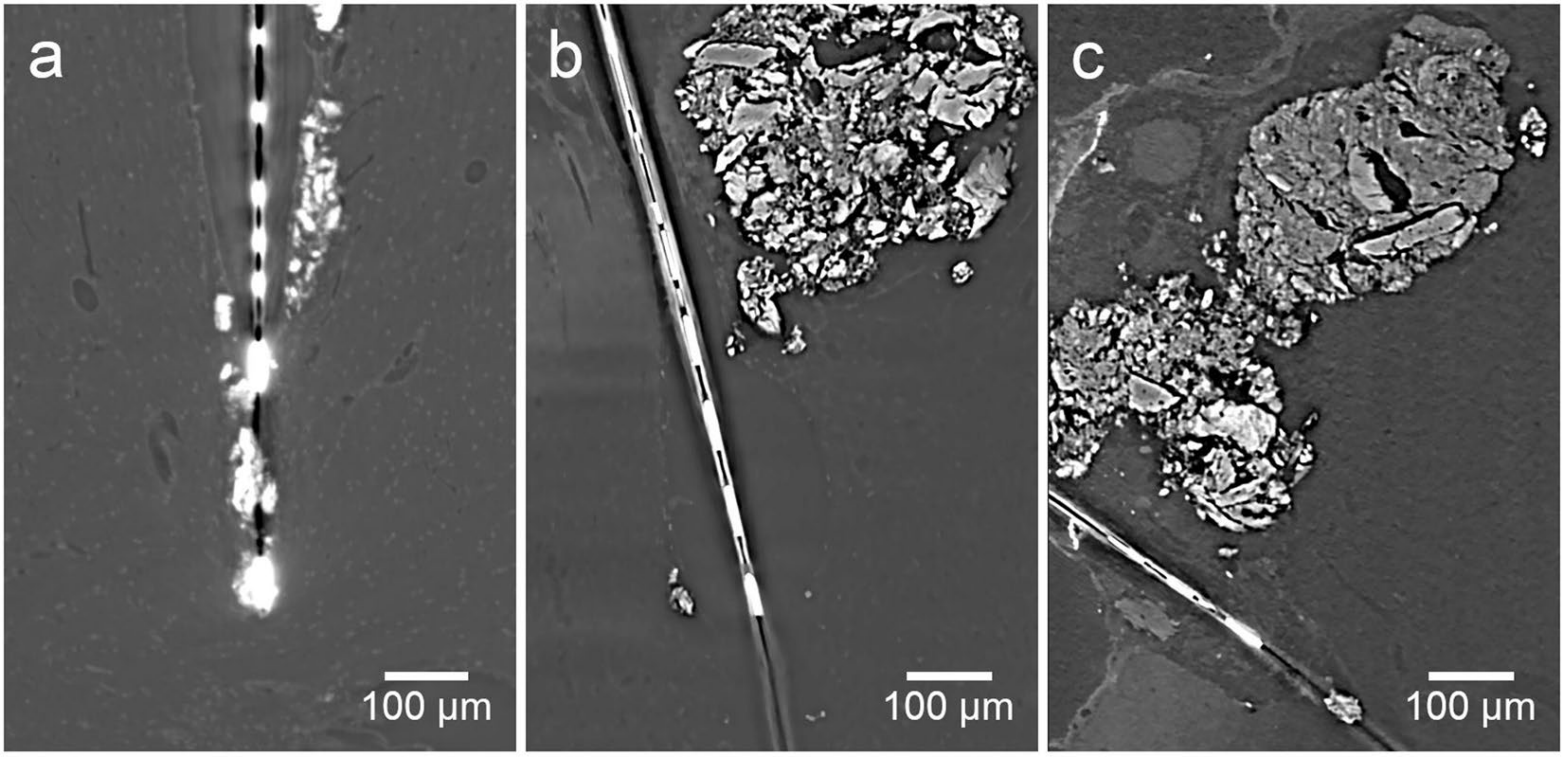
Virtual sections of X-ray tomograms with calcifications in close vicinity to implanted neural probes. Depicted are the tissue-probe interfaces of the 12 wpi sample (a), 2 wpi sample a (b), and 2 wpi sample b (c). Bright areas surrounding the probe represent calcifications that provide a significantly higher X-ray contrast than brain tissue.
